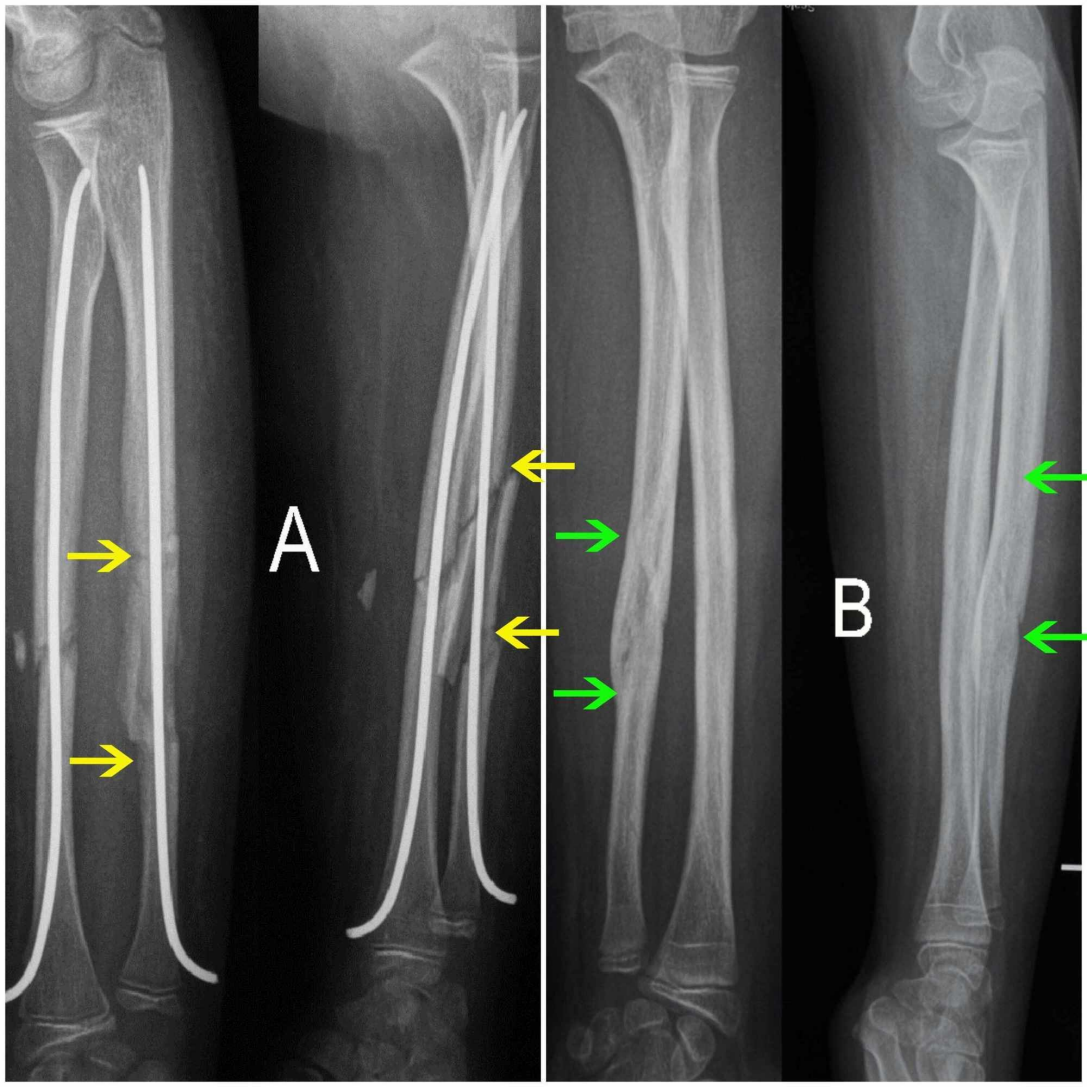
Ulnar segmental fracture Postoperative anteroposterior (AP) and lateral (L) X-ray of ulnar segmental fracture (yellow arrows) in both bone forearm fracture treated by ESIN. (B) Final follow-up AP and L X-ray after hardware removal showing complete healing of the ulnar fracture (green arrows), which is hardly evident anymore.
